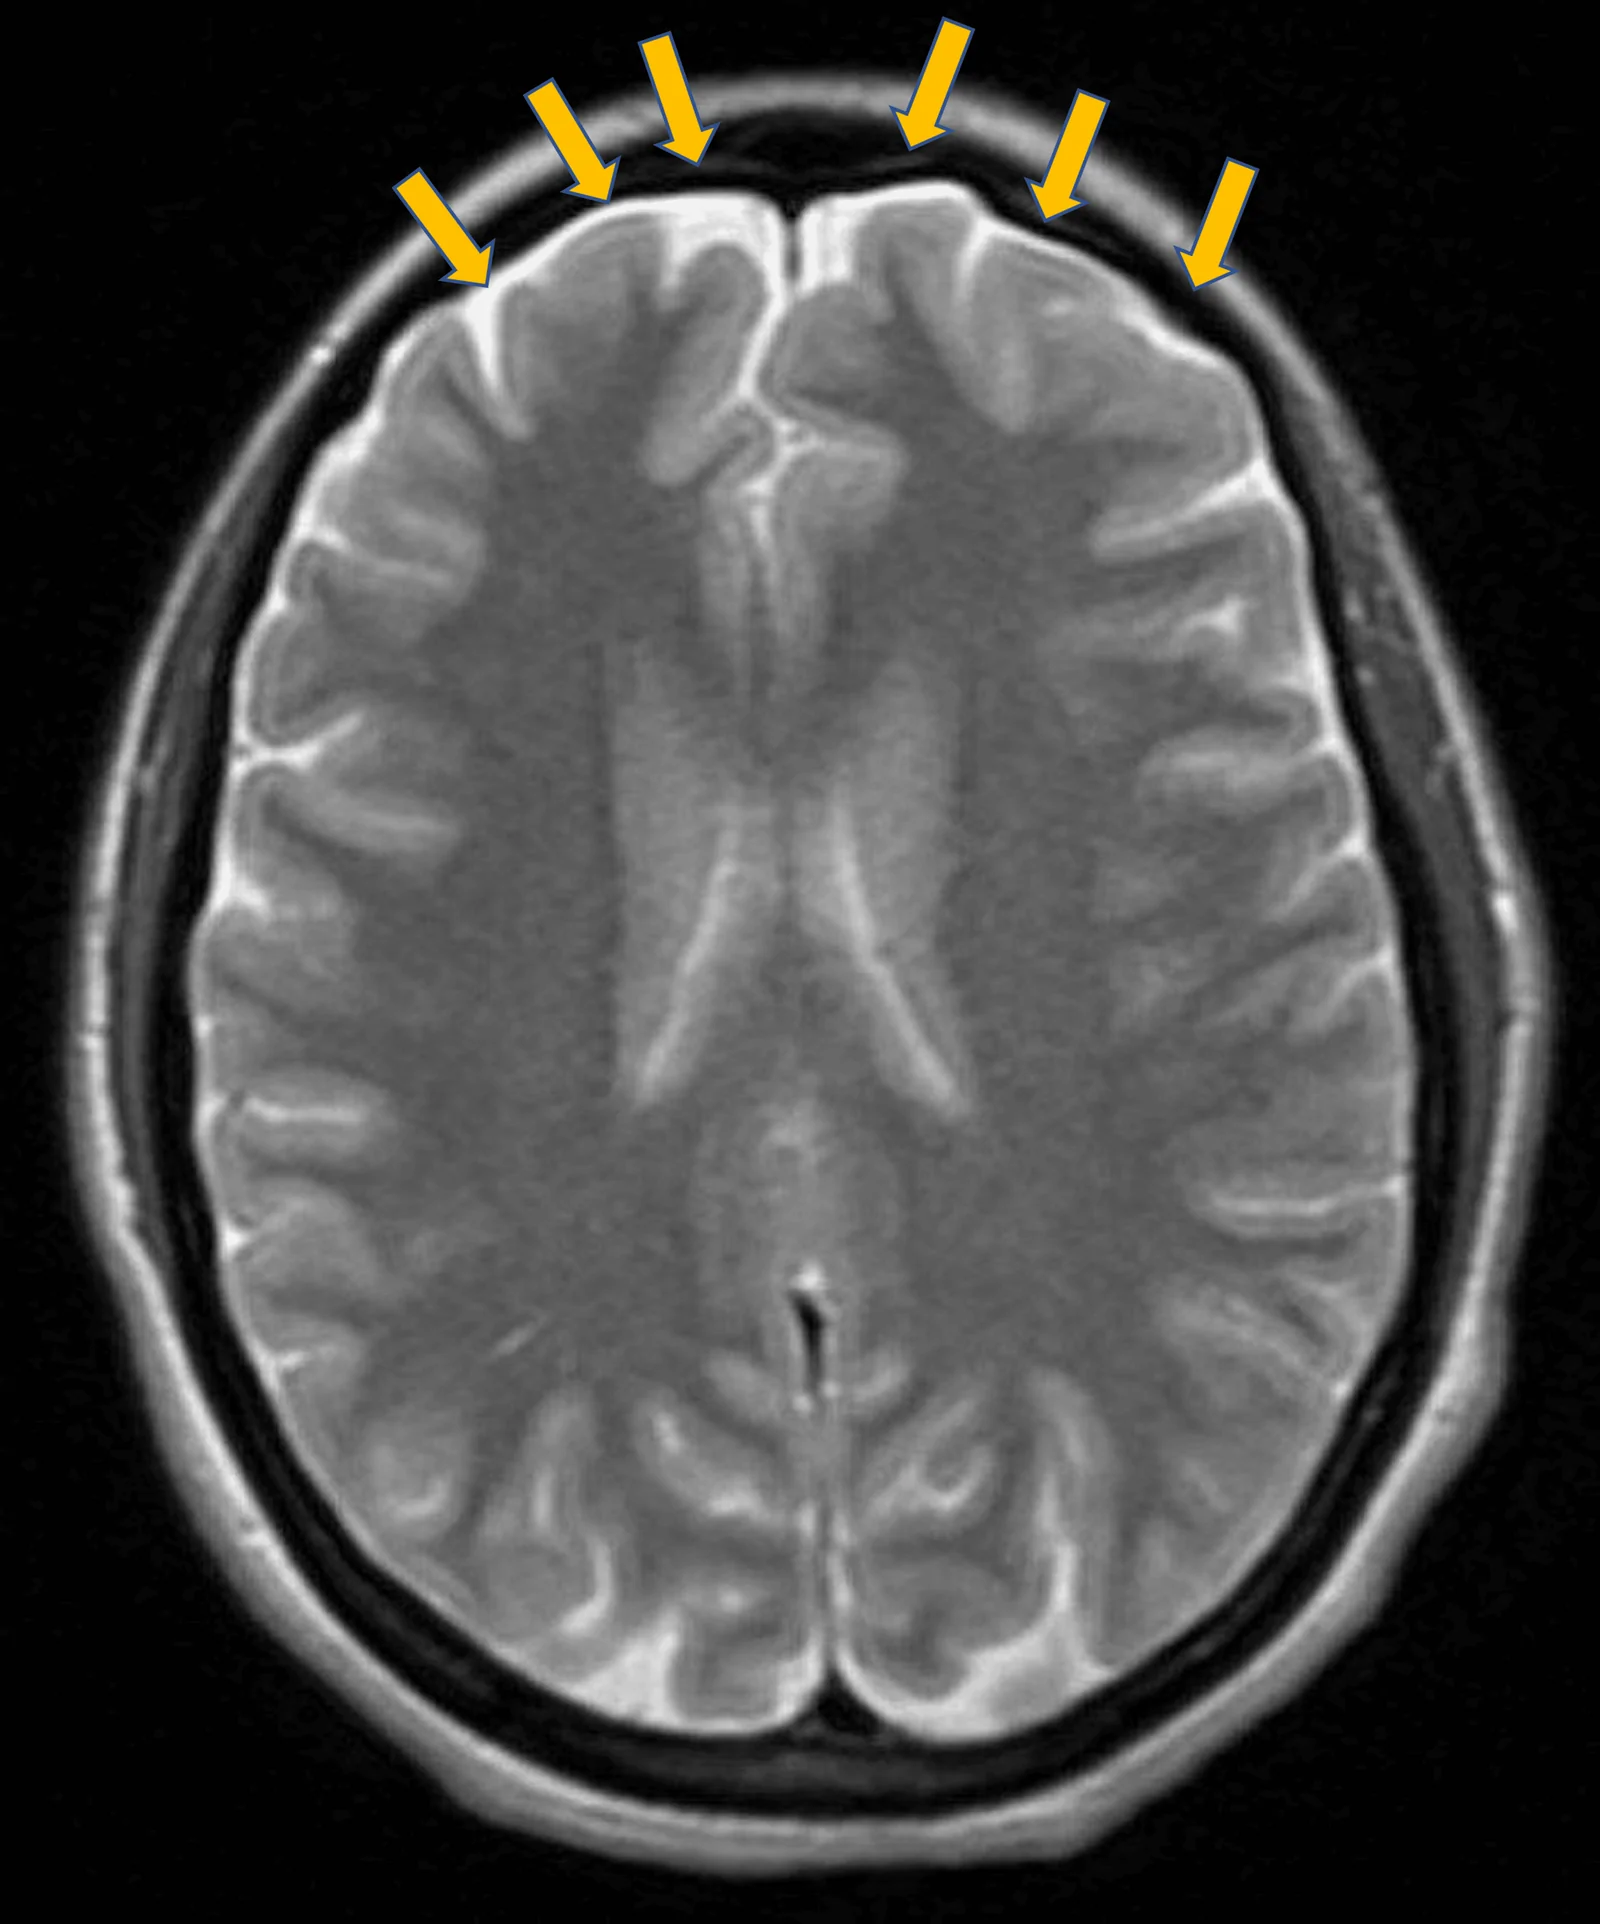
Acute brain MRI, T2 axial (Day 2). Orange arrows: expanded extra-axial CSF spaces at the frontoparietal convexities. No acute infarction, hemorrhage, or abnormal enhancement; ventricular system normal with no midline shift.
